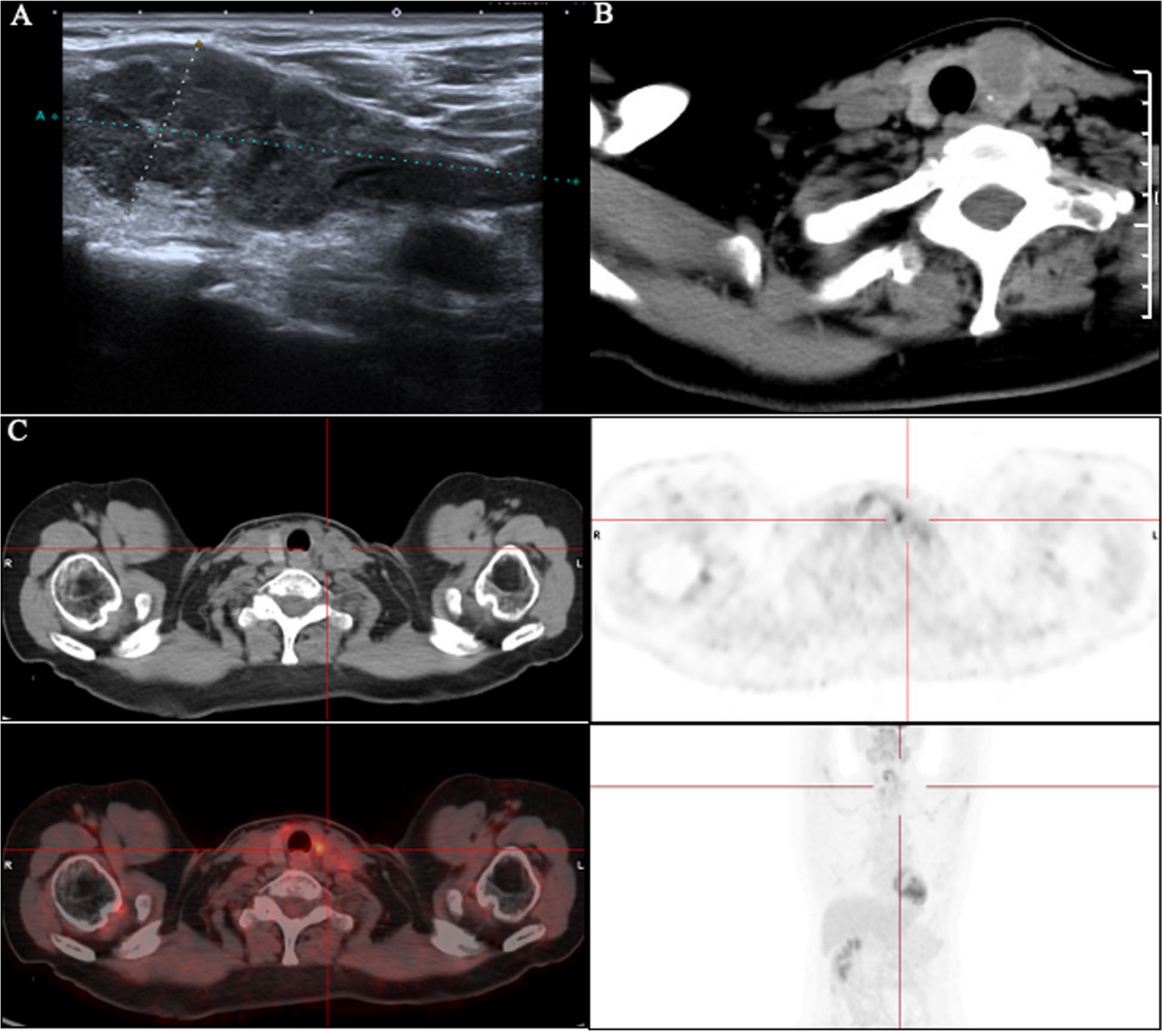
Abnormal imaging findings of patients. A The hypoechoic nodule grew horizontally and was composed of fused lesions, measuring 61.6 × 21.4 mm. B The preoperative computed tomography (CT) revealed bilateral thyroid nodules with unclear boundaries. C Three weeks after the surgery, positron emission tomography-CT revealed increased metabolism in the surgical site of the thyroid
